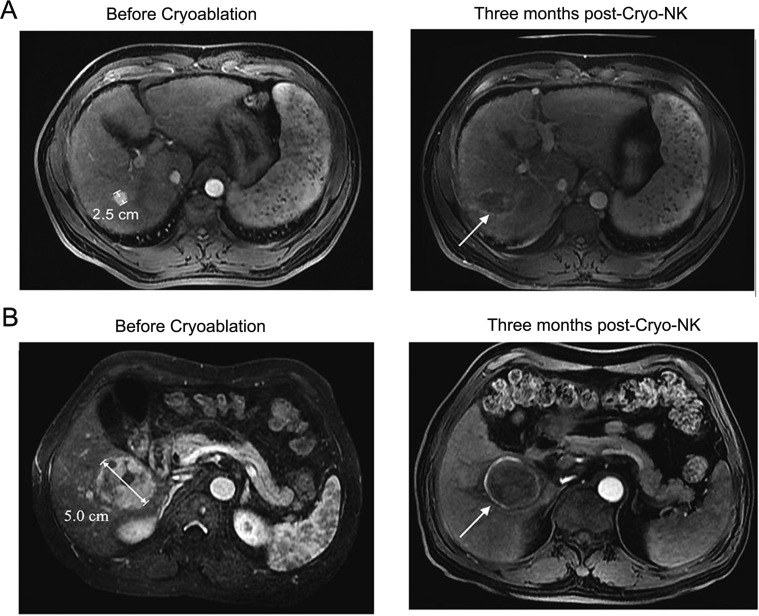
MRI images of 2 representative cases achieved CR at three months post-Cryo-NK (A) Case #1, a 50-year-old male, stage III, a maximum HCC nodule of 2.5 cm, MRI showed no enhancement in the occupying lesion, with mild shrinkage of the area; (B) Case #2, a 48-year-old female, stage IV, a maximum HCC nodule of 5.0 cm, MRI showed a lesion with a large area of necrosis.
